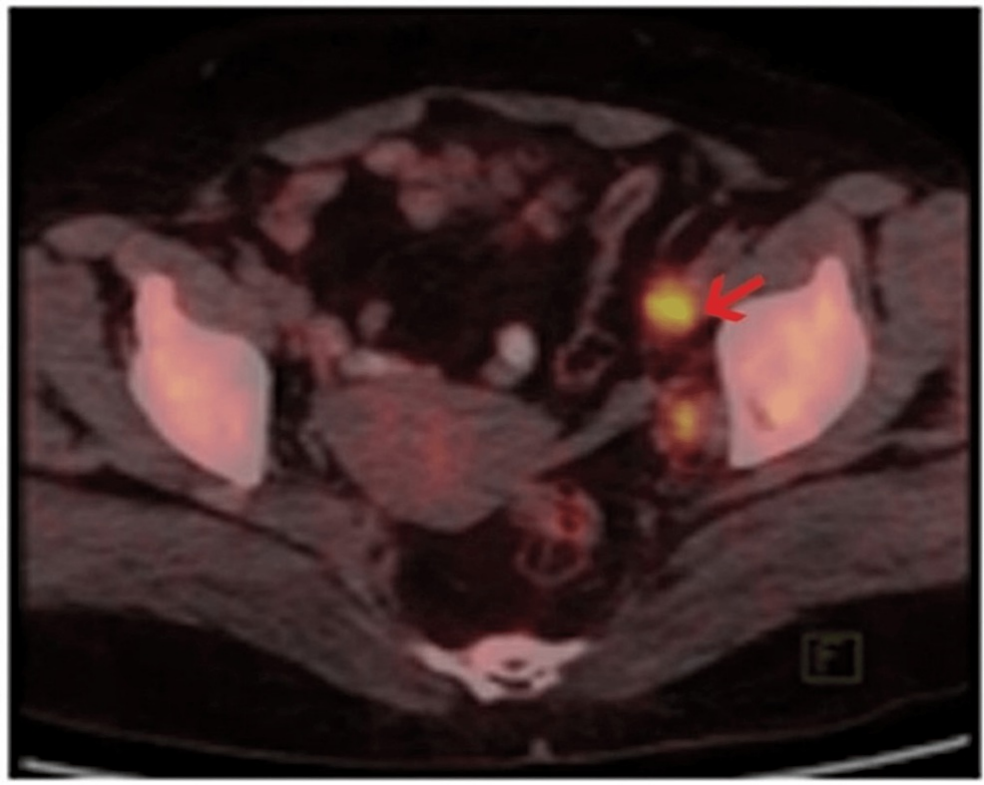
The PET/CT image showing left pelvic lymph node involvement The red arrow indicates FDG uptake of the left pelvic lymph node of an early cervical cancer patient. PET/CT: Positron emission tomography/computed tomography, FDG: Fluoro- D-glucose
